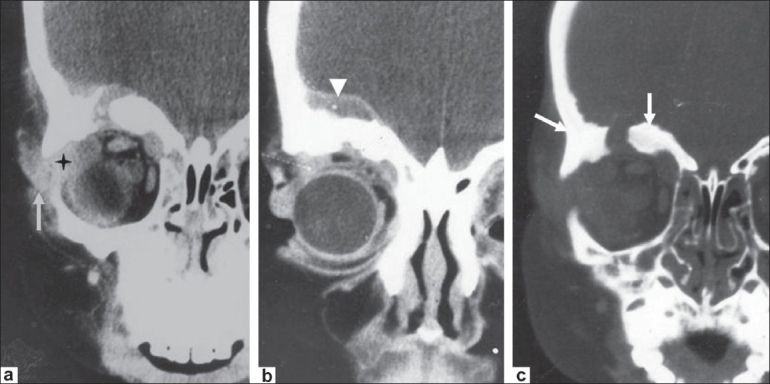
A 7-year-old boy presented with unilateral, painless, nonpulsatile proptosis of the right eye of 2 months' duration. Coronal contrast enhanced CT scans (a,b) of the orbit, show a hypodense mass in the lacrimal region (asterisk in a) with extension into the infratemporal fossa (arrow in a) and also intracranially (extradural abscess) (arrowhead in b). Coronal CT scan (c), shows associated destruction of the orbital plate of the frontal and zygomatic bones (arrows). The lateral rectus cannot be defined separately.
